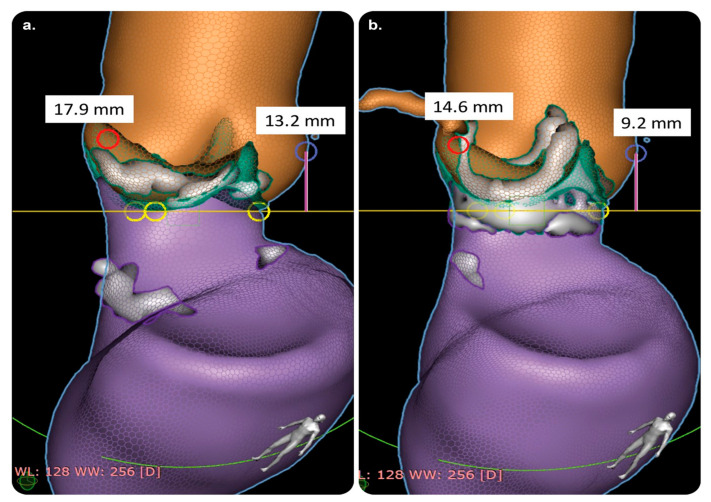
HeartNavigator software and its role in intuitive display. CT assessment of coronary heights before and after aortic valve replacement with implantation of the ‘RD-AV EDWARDS INTUITY’ system. (a) Preoperative RCAA and LCAA heights (red and blue circles, respectively; distance from the annular plane to the left coronary ostium). (b) Postoperative RCAA and LCAA heights (red and blue circles, respectively; distance from the annular plane to the right coronary ostium). Abbreviations: CT, computed tomography; LCAA: left coronary artery-to-annulus; RCAA: right coronary artery-to-annulus. Reprinted from “Effect of conventional and rapid-deployment aortic valve replacement on the distance from the aortic annulus to coronary arteries” by Coti et al. [60] with written approval from the corresponding author and publisher (Interactive CardioVascular and Thoracic Surgery; Copyright 2021).
